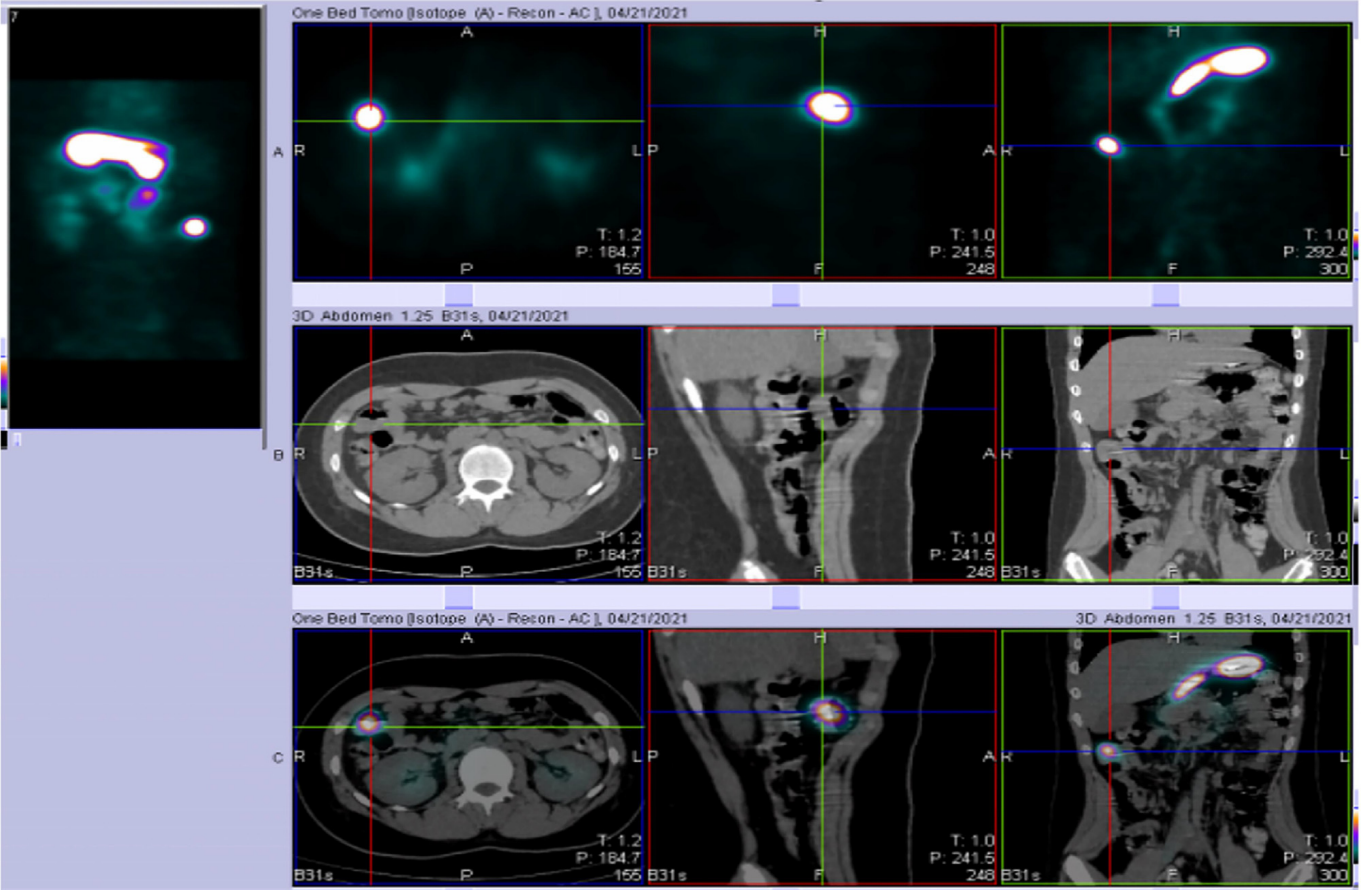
SPECT/CT fusion imaging revealed a focal radiotracer accumulation within the right upper hemiabdomen localized to an apparent blind-ending loop of bowel in the small intestine.
